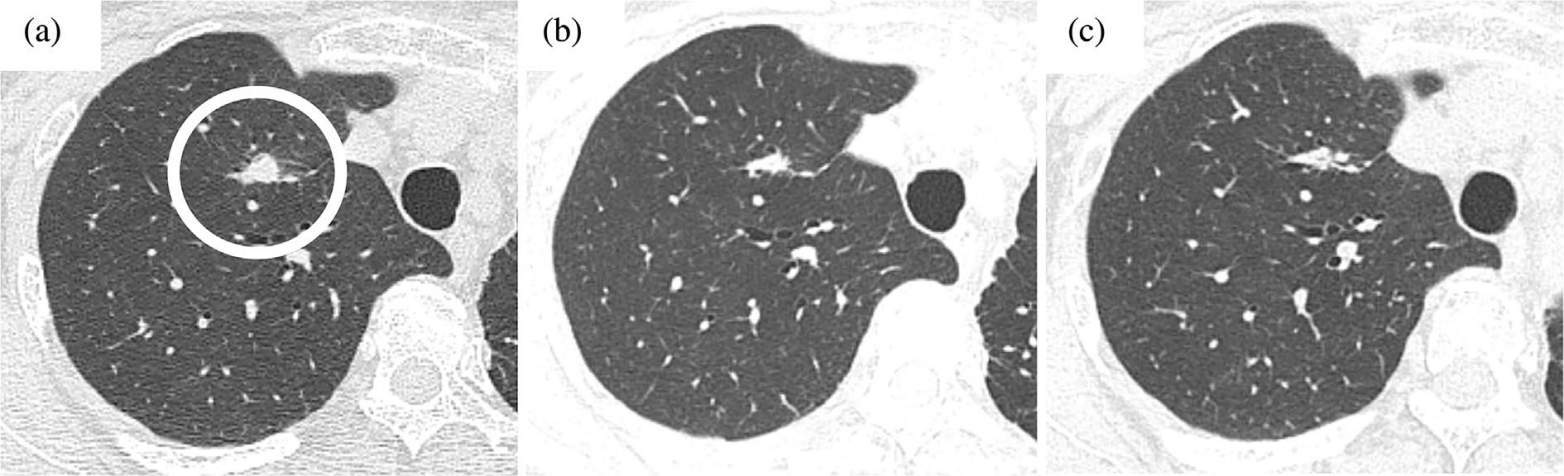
(a) Chest computed tomography (CT) showed a recurrent lesion in the right upper lobe (circle) after 2 years post‐surgery. Combination therapy with erlotinib plus ramucirumab resulted in tumor shrinkage after (b) 3 months and (c) 16 months.
